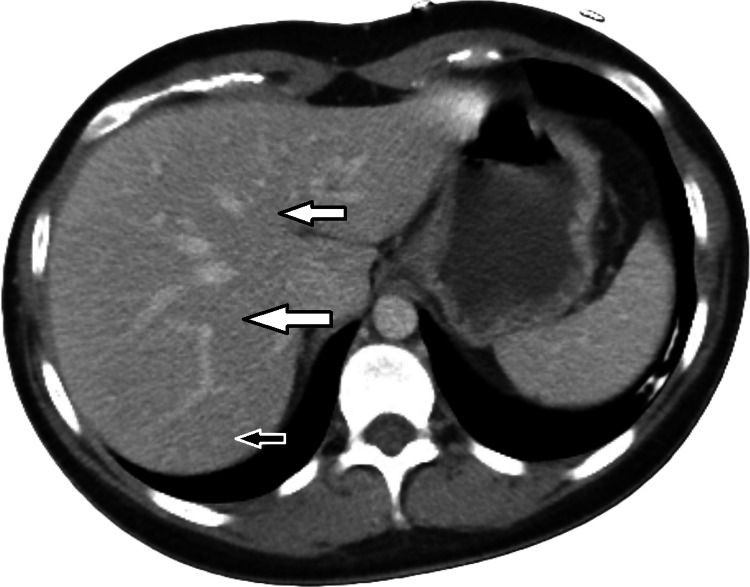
Computed tomography (CT) abdomen and pelvis with IV contrast Computed tomography (CT) abdomen and pelvis with intravenous contrast shows normal hepatic biliary ducts without dilatation (arrows).
